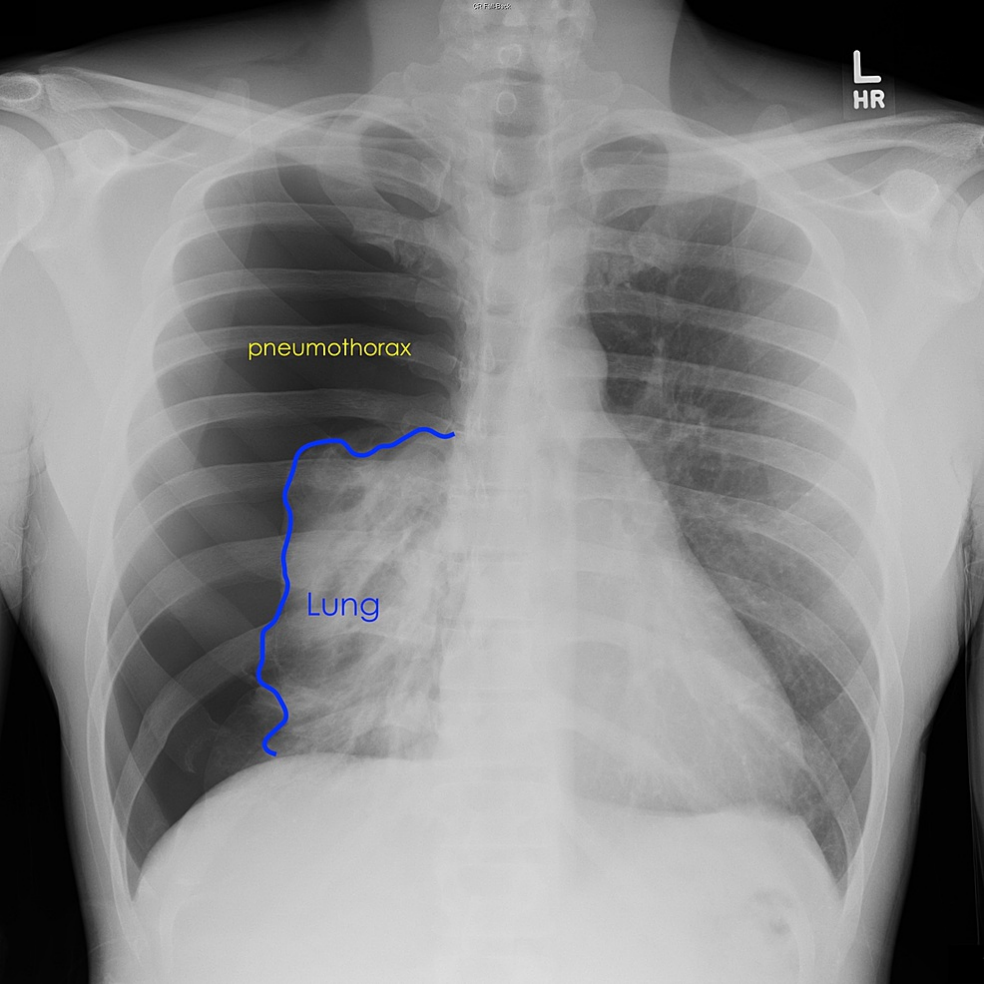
Chest radiograph demonstrating a 90% right-sided pneumothorax
